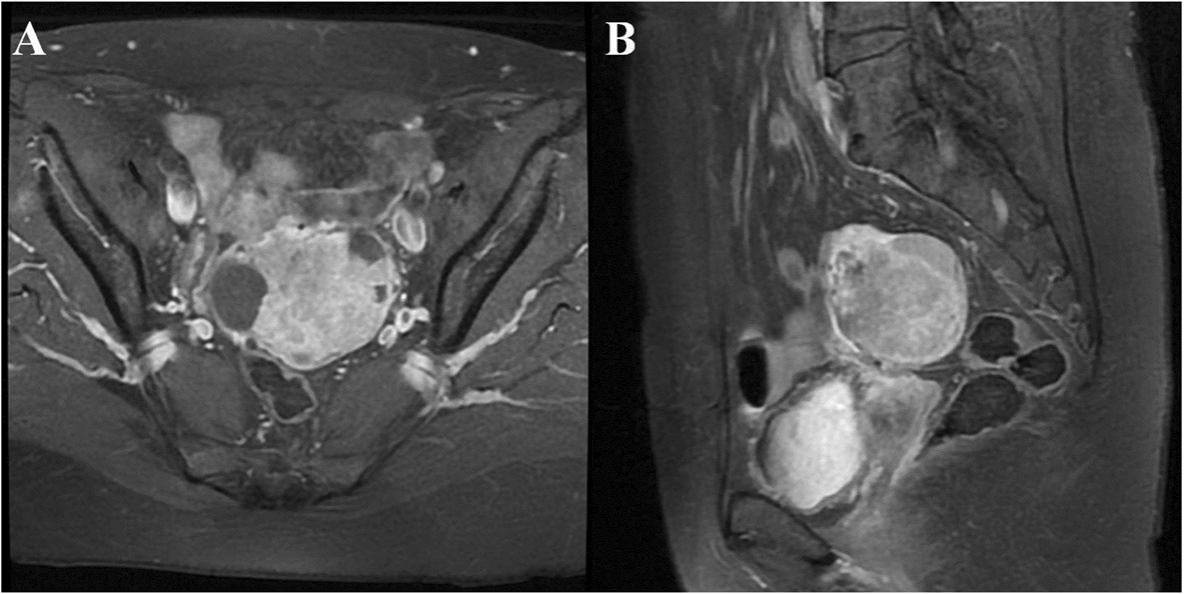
Pelvic magnetic resonance. A) Axial fat suppressed T1-weighted image after intravenous gadolinium enhancement. B) Sagittal T2-weighted image showing left solid ovarian mass.
